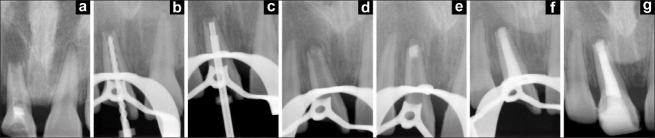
Series of radiographs showing placement of the barrier material and mineral trioxide aggregate. (a) Pre-operative radiograph showing remnants of calcium hydroxide paste; (b) 140 no. K-file placed to working length; (c) System of needles used as a carrier for placement of the barrier material and mineral trioxide aggregate; (d) Barrier material in place in area of the bony lesion; (e) Mineral trioxide aggregate compacted against the barrier material; (f) Backfill performed using thermoplasticized Gutta-percha in the next appointment; (g) Completed coronal restoration
